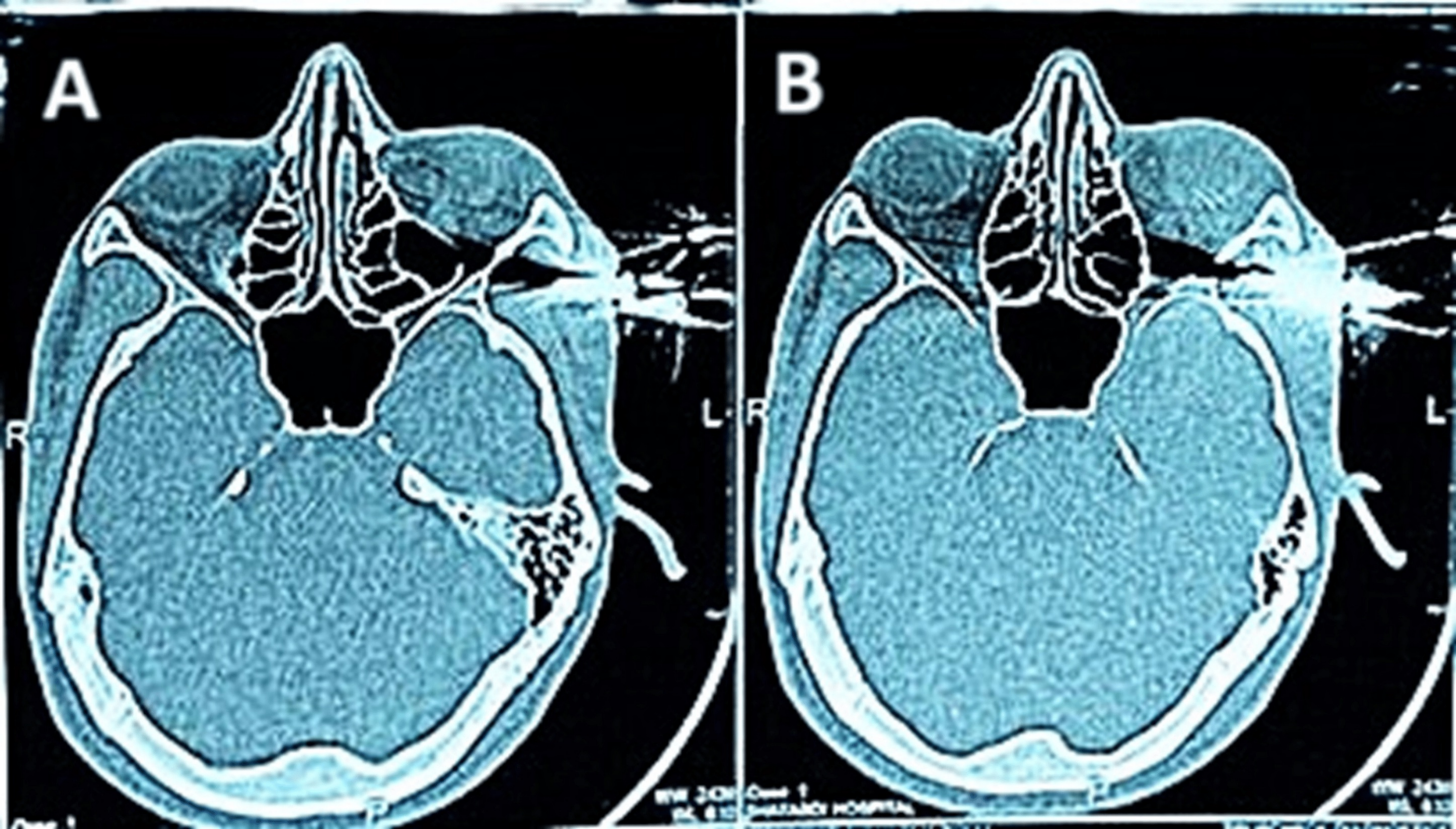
Computed tomography of Brain. (A) Metallic foreign body; (B) Metallic artifacts of foreign body and no intracranial penetration.
